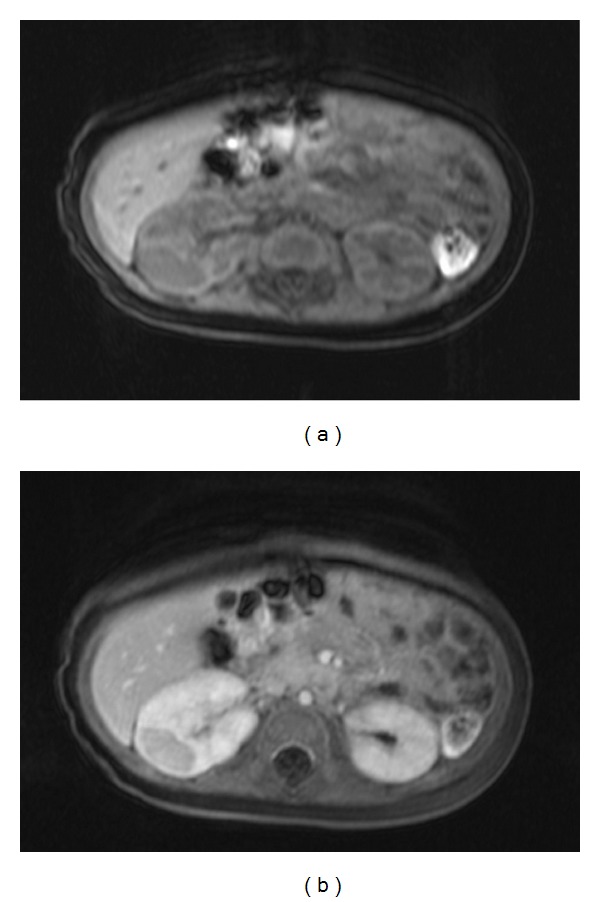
Noncontrast (a) and contrast enhanced (b) T1 weighted MR images show a large hypointense cortical mass at the right kidney and multiple smaller foci in both kidneys.
